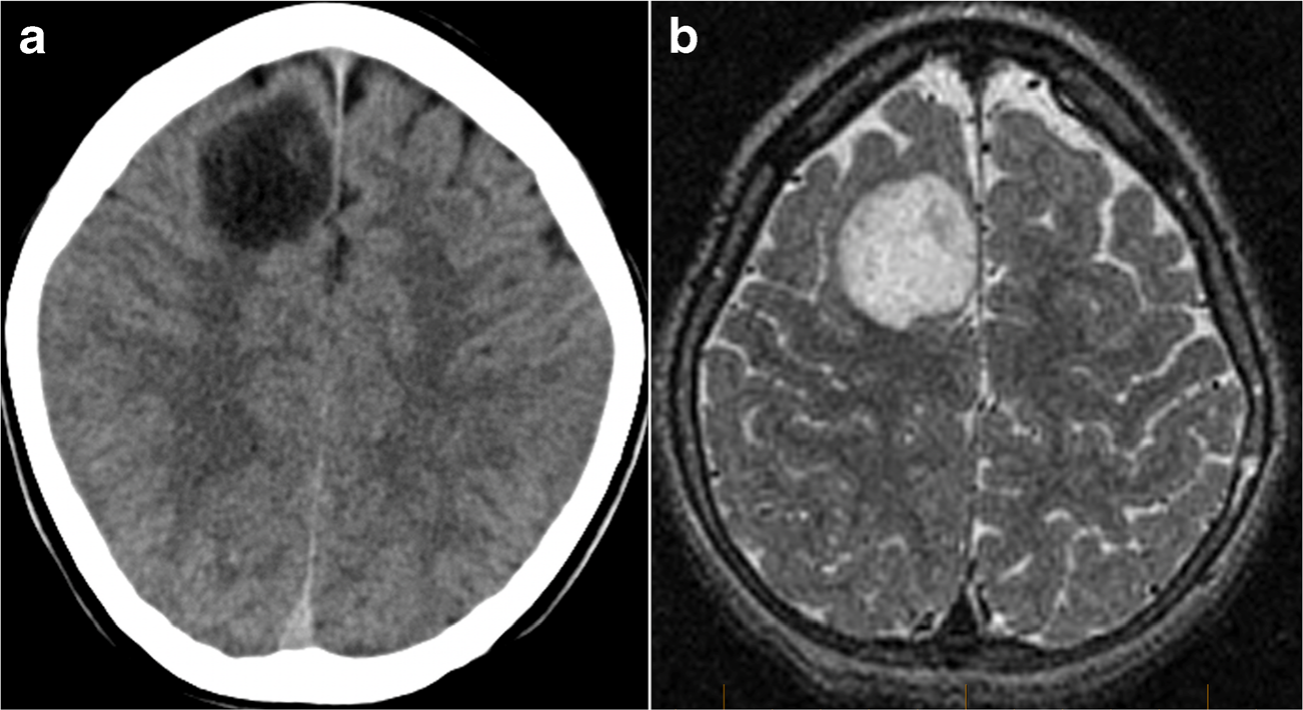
Isomorphic diffuse glioma. a Axial non-contrast CT scan through the frontal lobes shows a well-circumscribed hypodense tumor in the right frontal lobe. b Axial T2-weighted image through the same level demonstrates a well-circumscribed T2-hyperintense tumor
